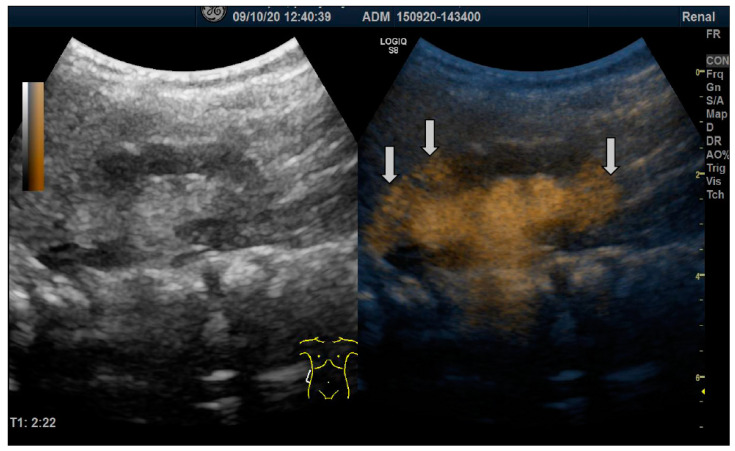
The duplex view of the right kidney. On the left side of the figure, the right kidney is visible in the B-mode. On the right side of the picture, the ultrasound contrast media is visible in the mildly dilated pyelo-caliceal system with convex calyces. In the upper and lower poles, the contrast media is visible in the renal parenchyma (arrows). The diagnosis is VUR grade III with intrarenal reflux in the upper and lower pole.
